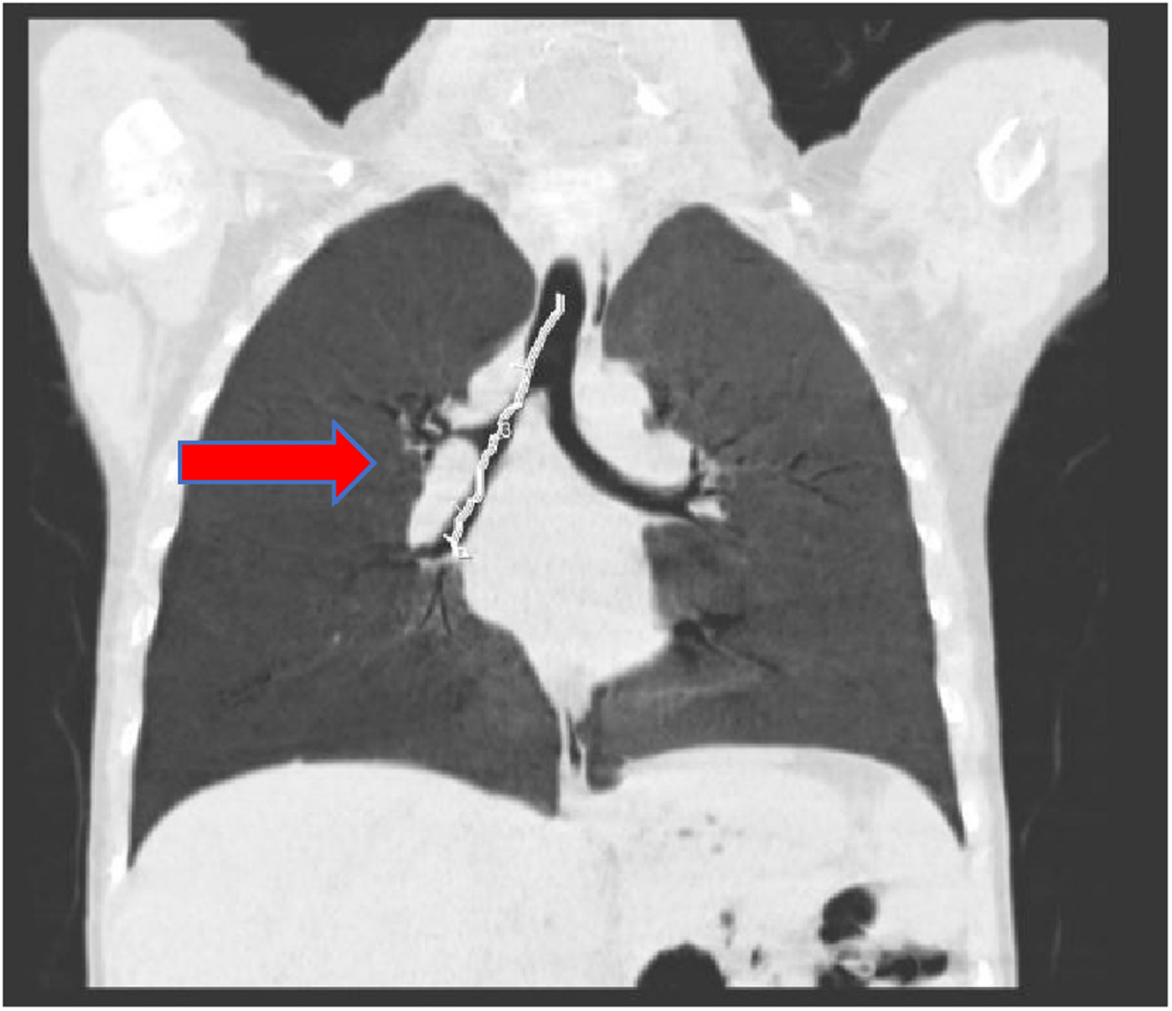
Partial obstruction of right main bronchus is remarked in the coronal cut of the chest CT scan
